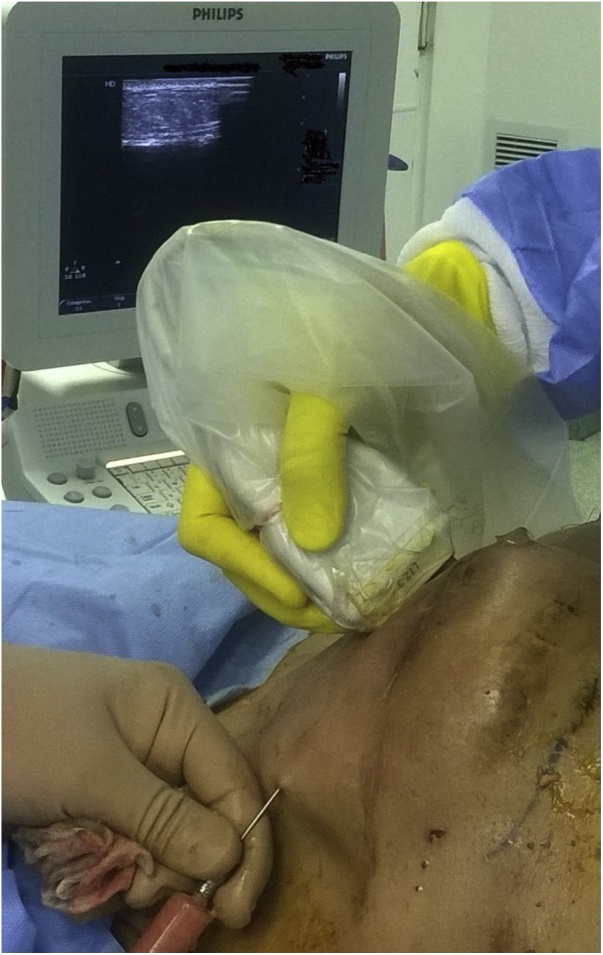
Ultrasound guided lipofilling.
